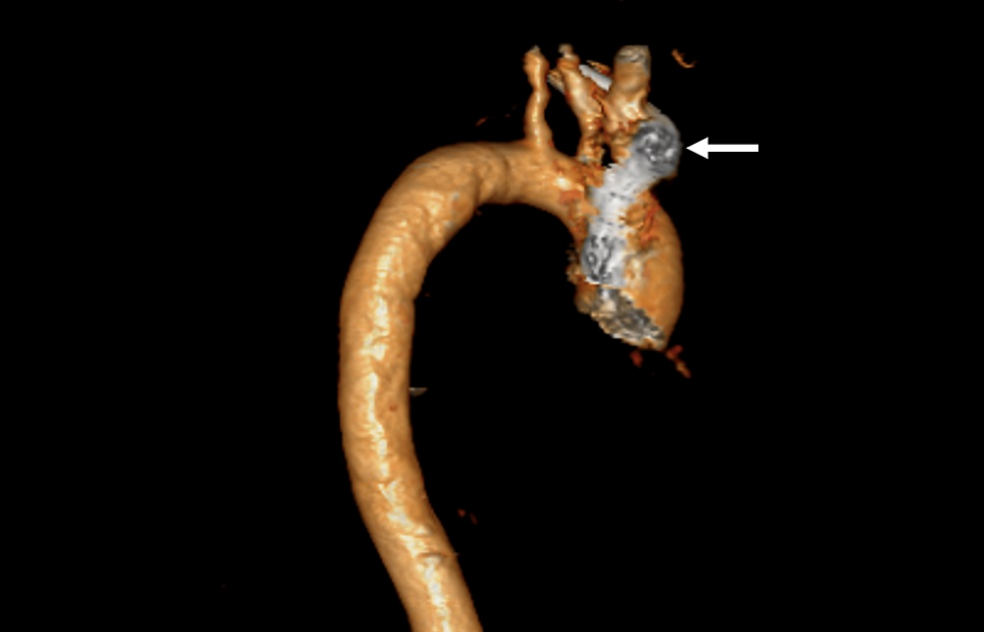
Preoperative CT-aortogram (3D) showing a dilated aorta with a periaortic mass lesion (arrow).
